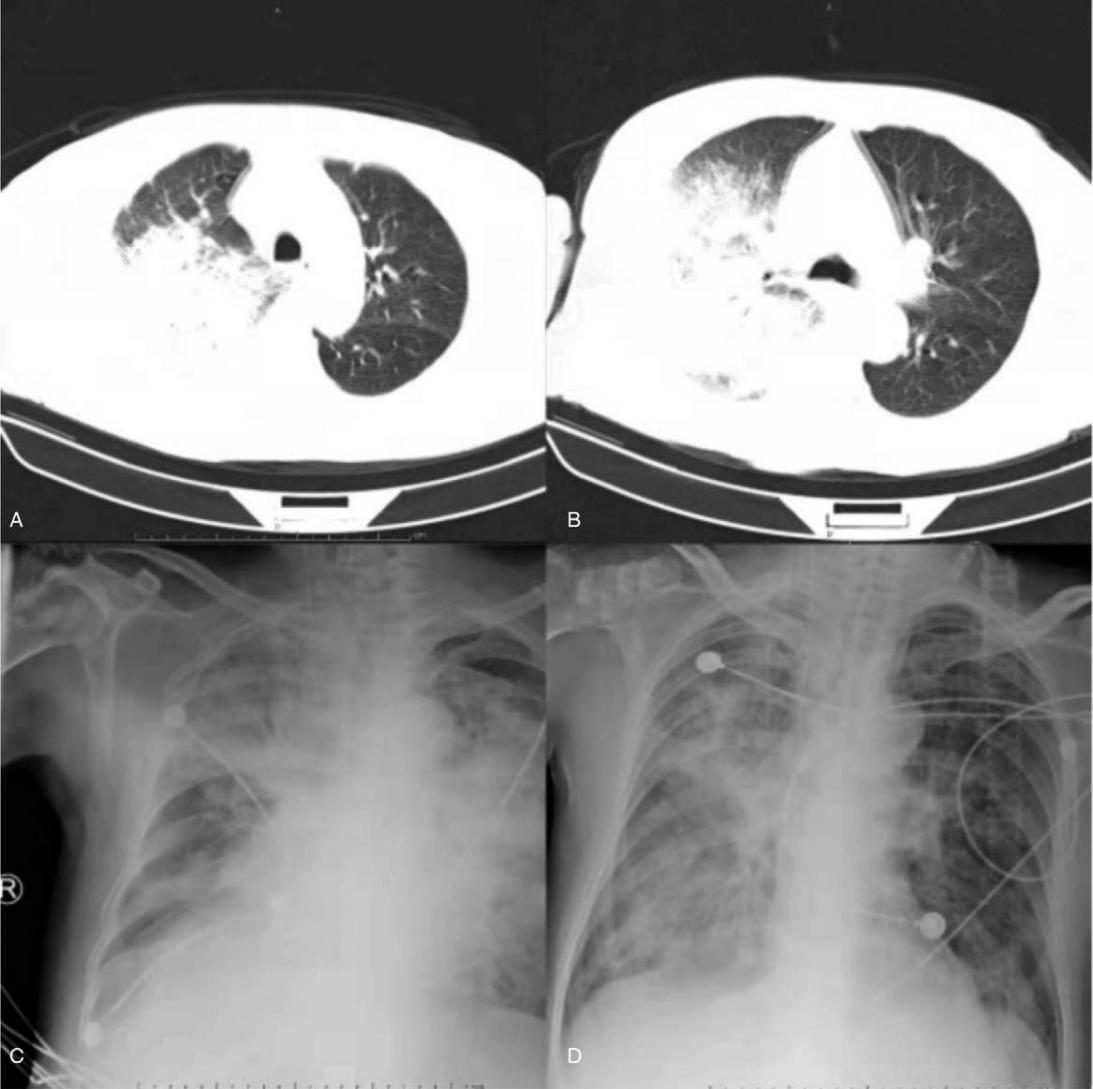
Chest CT and X-ray of the patient: A and B, On the day of admission, C, 4 days after doxycycline treatment, and D, 7 days after doxycycline treatment, suggesting that the lung infection improved.
